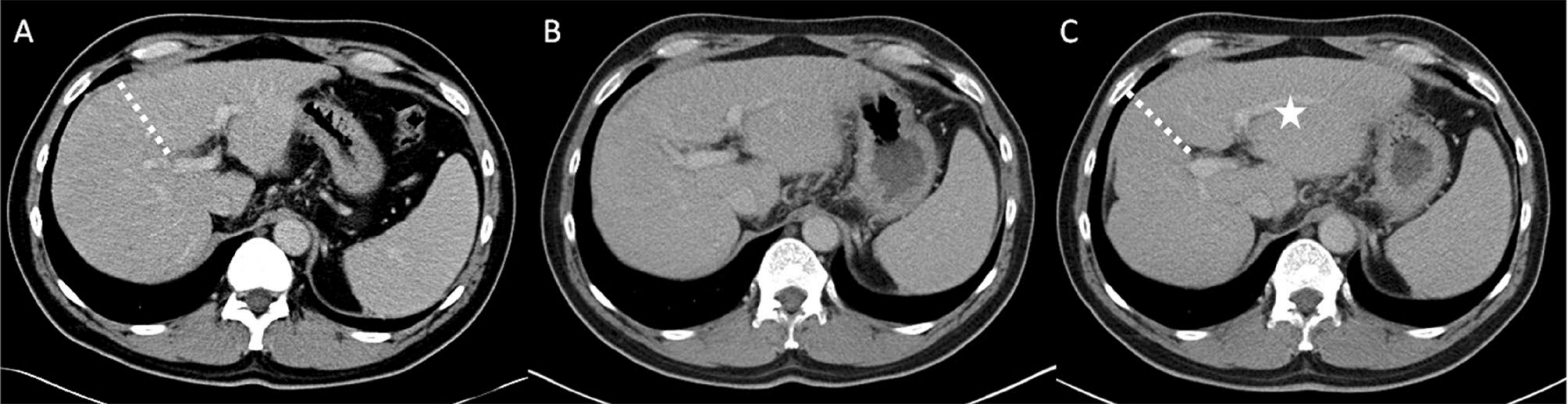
Hypertrophy of the left liver lobe after right-sided radioembolization. Computed tomography images (A) pre, (B) 3, and (C) 6 months after radioembolization. Significant volume increase of the left liver lobe (*) can be observed post-therapy. Dotted line: border between left and right liver lobe.
